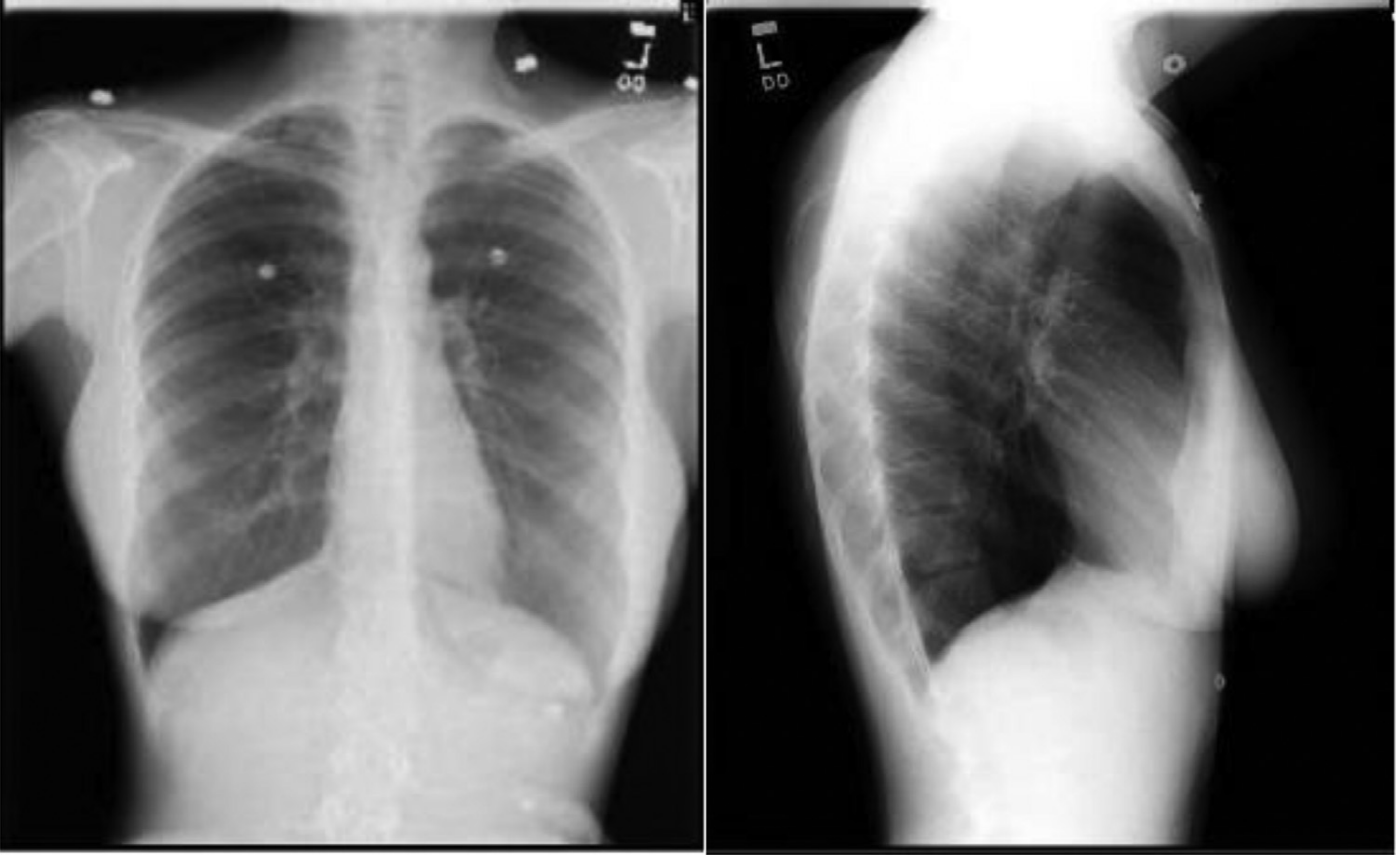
Chest X-ray AP and lateral views - looks within normal limits without any evidence of pneumothorax, effusion or atelectasis.
